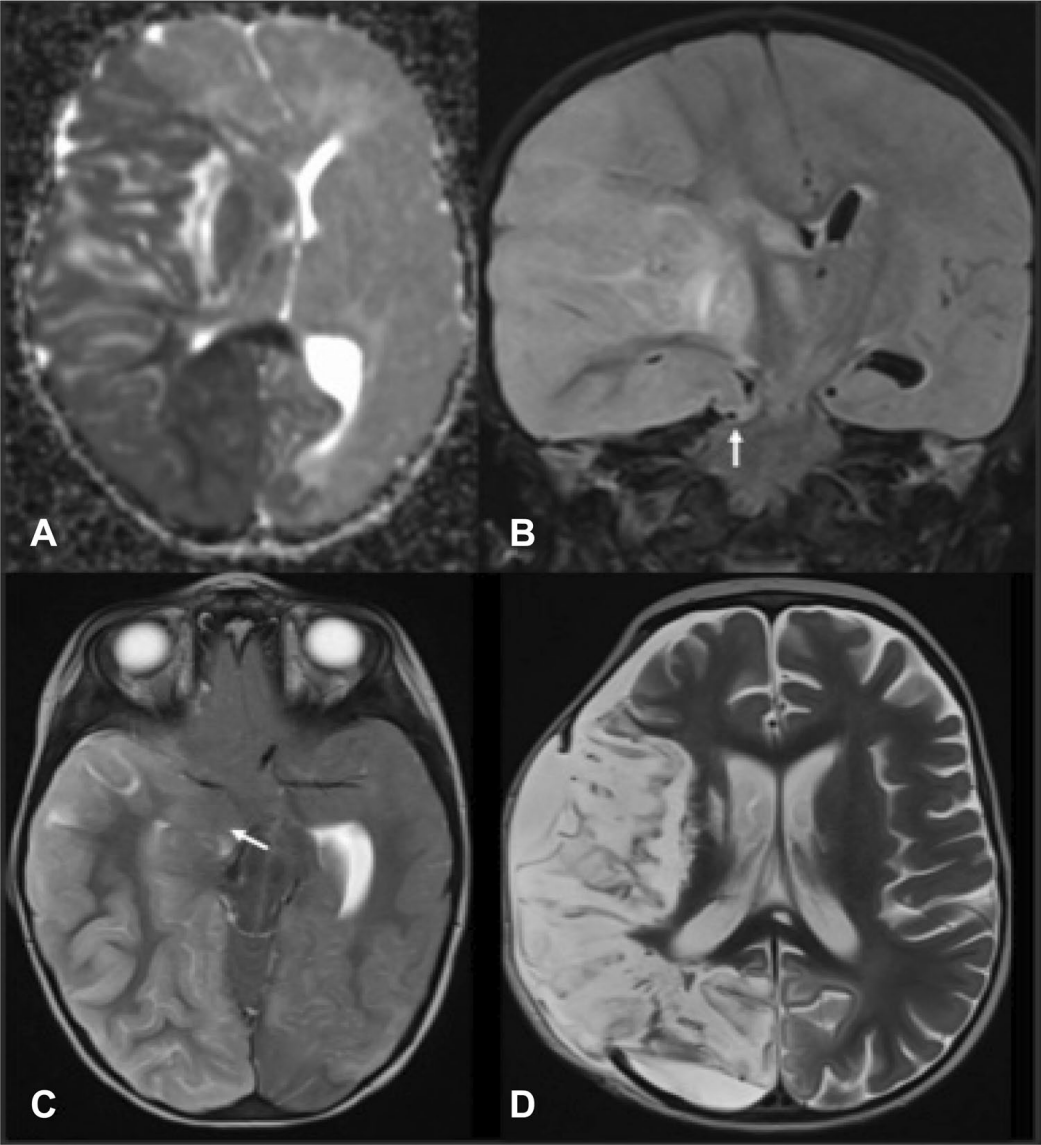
Anisotropy diffusion coefficient (ADC) map. Initial MRI shows a large subacute ischemic lesion on the right MCA and PCA territory. Restricted diffusion of the splenium and contralateral occipital mesial cortex involvement (A). Coronal T2 FLAIR and axial T2. Deviation of the midline with transtentorial herniation of the frontobasal parenchyma, signs of uncal herniation with effacement of the interpeduncular cistern, and severe mass effect on the midbrain (*arrows*) are also evident (B, C). Follow-up MRI performed 4 weeks later shows an extensive cortico-subcortical volume loss of the affected parenchyma, including the right putamen. Focal lesions are depicted in the right thalamus and splenium. A minimal parenchymal herniation through the flap is also noted (D)
